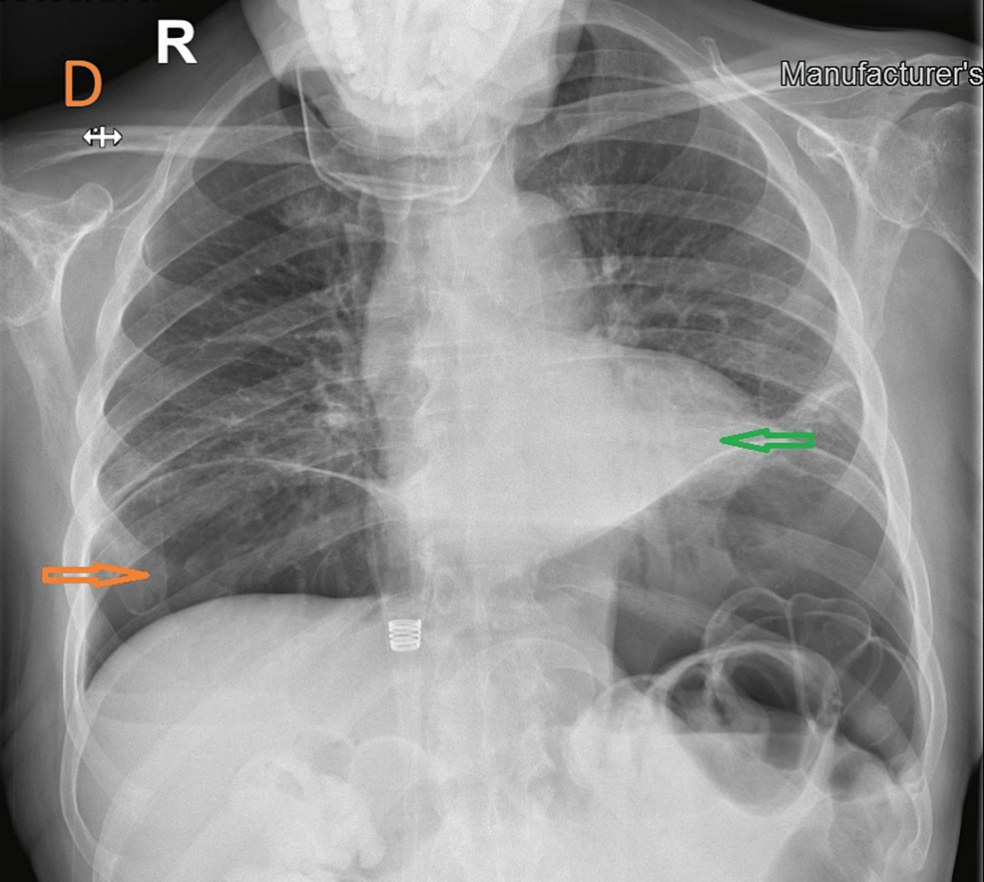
Upright Chest X-ray after paracentesis. Reduction in the size of the pneumoperitoneum and partial resolution of lobar collapse is observed.
